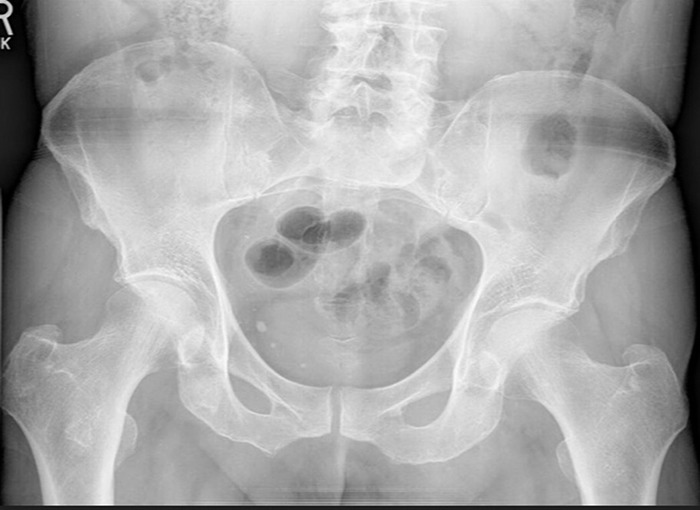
Preoperative anteroposterior radiograph shows mild spurring of bilateral acetabulae with mild joint space narrowing.
